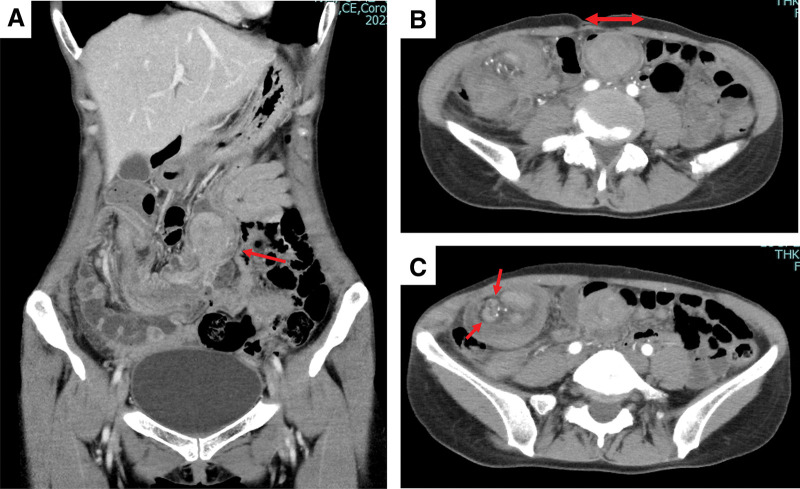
Contrast-enhanced CT scan of the abdomen (A) Superimposition of the ascending colon and the end of the ileum within the transverse colon. (B) 34 mm-sized mass in the advanced part of the colon. (C) Large intestinal peritoneal lymph nodes are observed.
